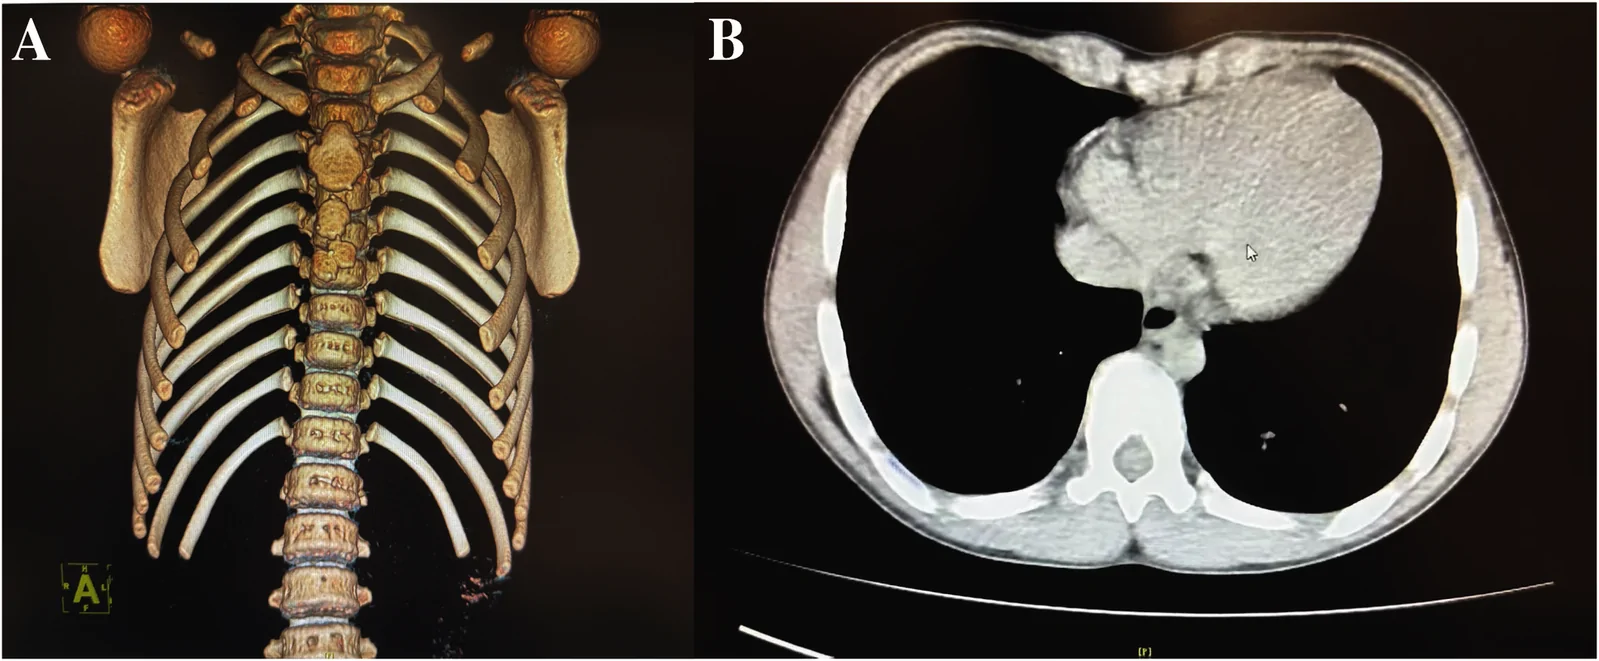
Radiological features of the chest of CCD. (A) Three-dimensional CT reconstruction demonstrates bilateral clavicular hypoplasia with segmental discontinuity, consistent with pseudoarthrosis. The clavicles appear shortened with dysplastic morphology, while the shoulder girdle remains relatively preserved. (B) Axial chest CT shows mild sternal depression without evidence of cardiopulmonary compression.
